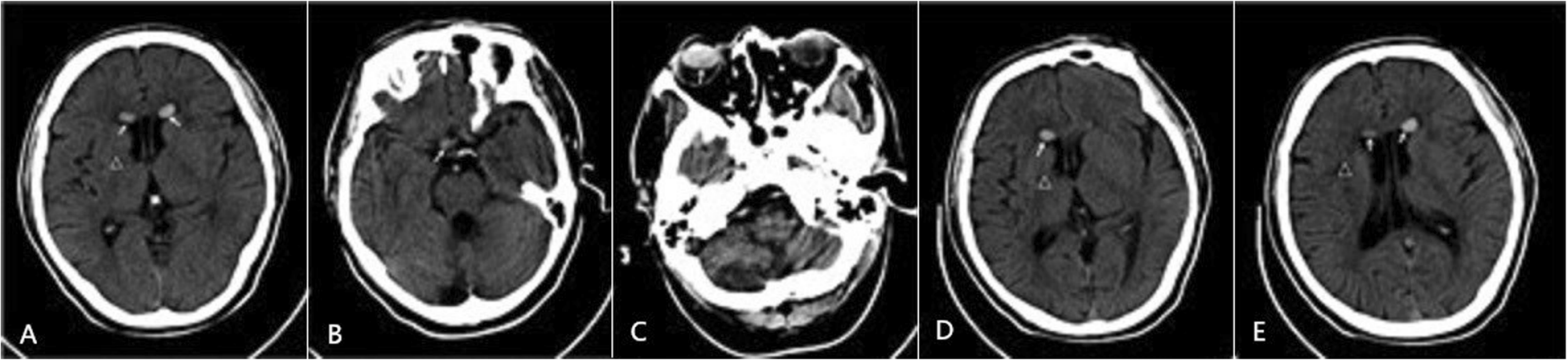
A 63-year-old male undergoes CT examination in December 2011 (ww 60, wl 100). a ~ c show the high-density shadow at bilateral ventricular anterior horns, right of suprasellar cistern and right eyeball, respectively (arrow). d and e display that the location of the high-density shadow in bilateral lateral ventricular anterior horns remains unchanged after 6 h (arrow).The old infarction at right basal ganglia is shown in a, d and e (△)
